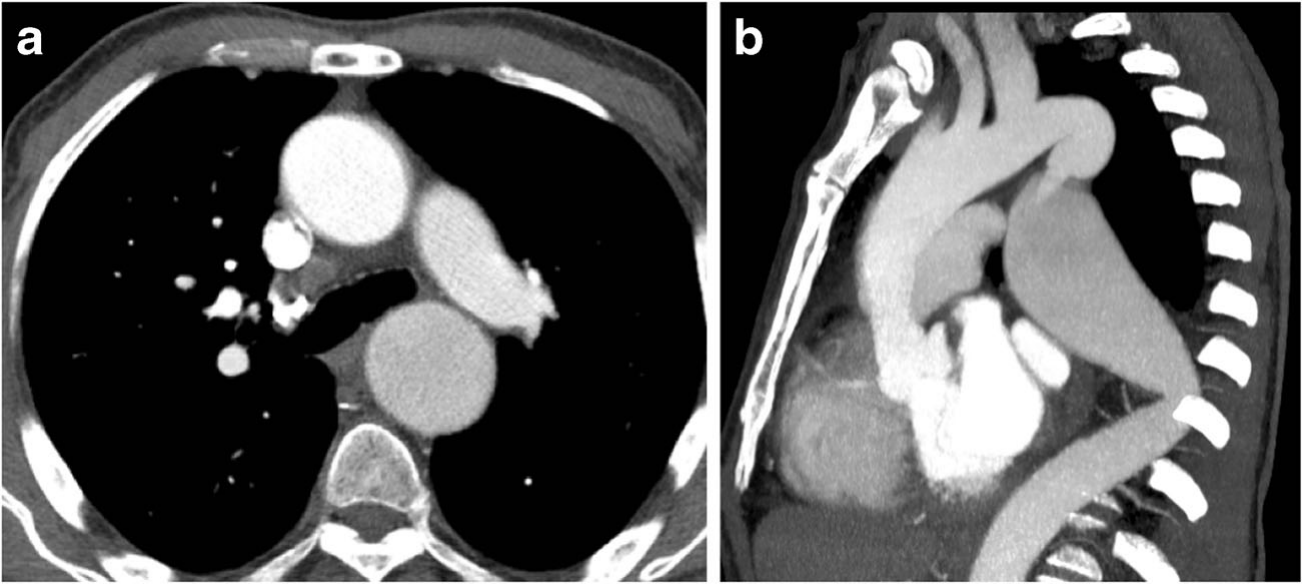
Axial CT image in 46-year-old patient with 20-year H/O essential hypertension presenting with chest pain being evaluated for aortic dissection demonstrates differential enhancement of the ascending and descending aorta due to severe stenosis from coarctation. Sagittal CECT MIP image demonstrates differential opacification of the aorta proximal and distal to the aortic coarctation with post-stenotic dilatation. Pressure gradient measured during catheter angiography was 20 mm Hg across the stenosis
